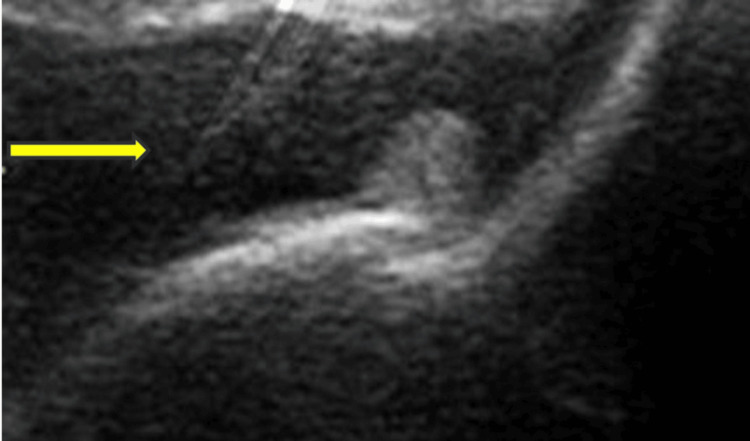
Ultrasonography (USG) of the ankle joint, anterior longitudinal view (synovial tissue) The yellow arrow indicates an anechoic area in the ankle joint.
